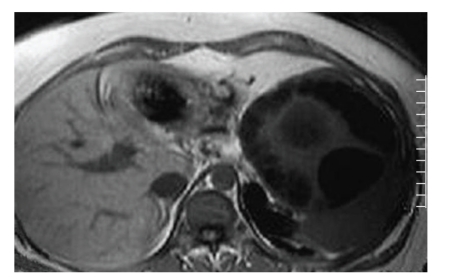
Axial noncontrast T1-weighted MRI shows multivesicular splenic cyst with low-signal-intensity.
